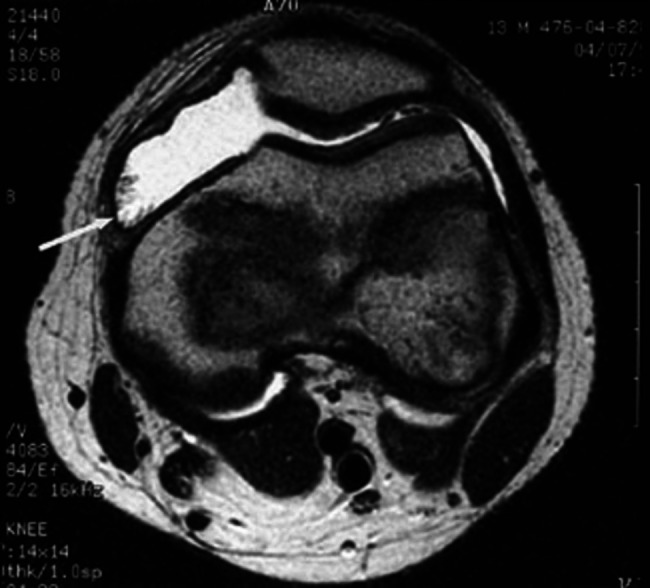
MRI of the right knee with proton density-weighted axial view showing hyperplasia of the synovium and low signal intensity indicating hemosiderin (arrow)
